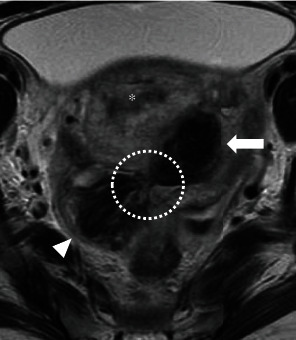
Magnetic resonance image on day two after embolization. Axial T2-weighted magnetic resonance image shows a hematoma within the broad ligament (arrow) adjacent to the postpartum uterus (asterisk) and hemoperitoneum (arrowhead). A continuous hematoma is observed from the thrombosed uterine artery pseudoaneurysm to the abdominal cavity, suggesting laceration of the left broad ligament (dotted circle).
